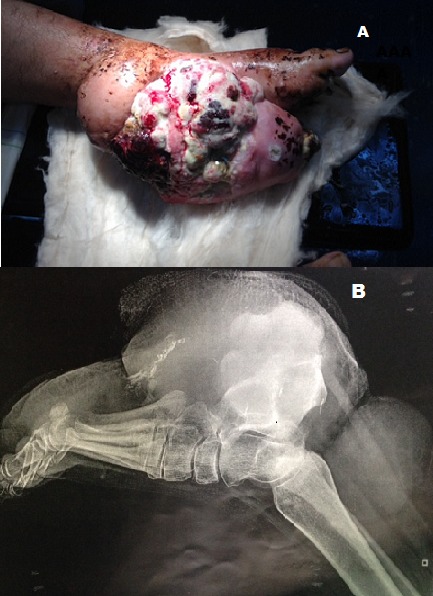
(A): hémangiopericytome malin du pied; (B): radiographie standard montrant l'extension de la tumeur vers le calcanéum
